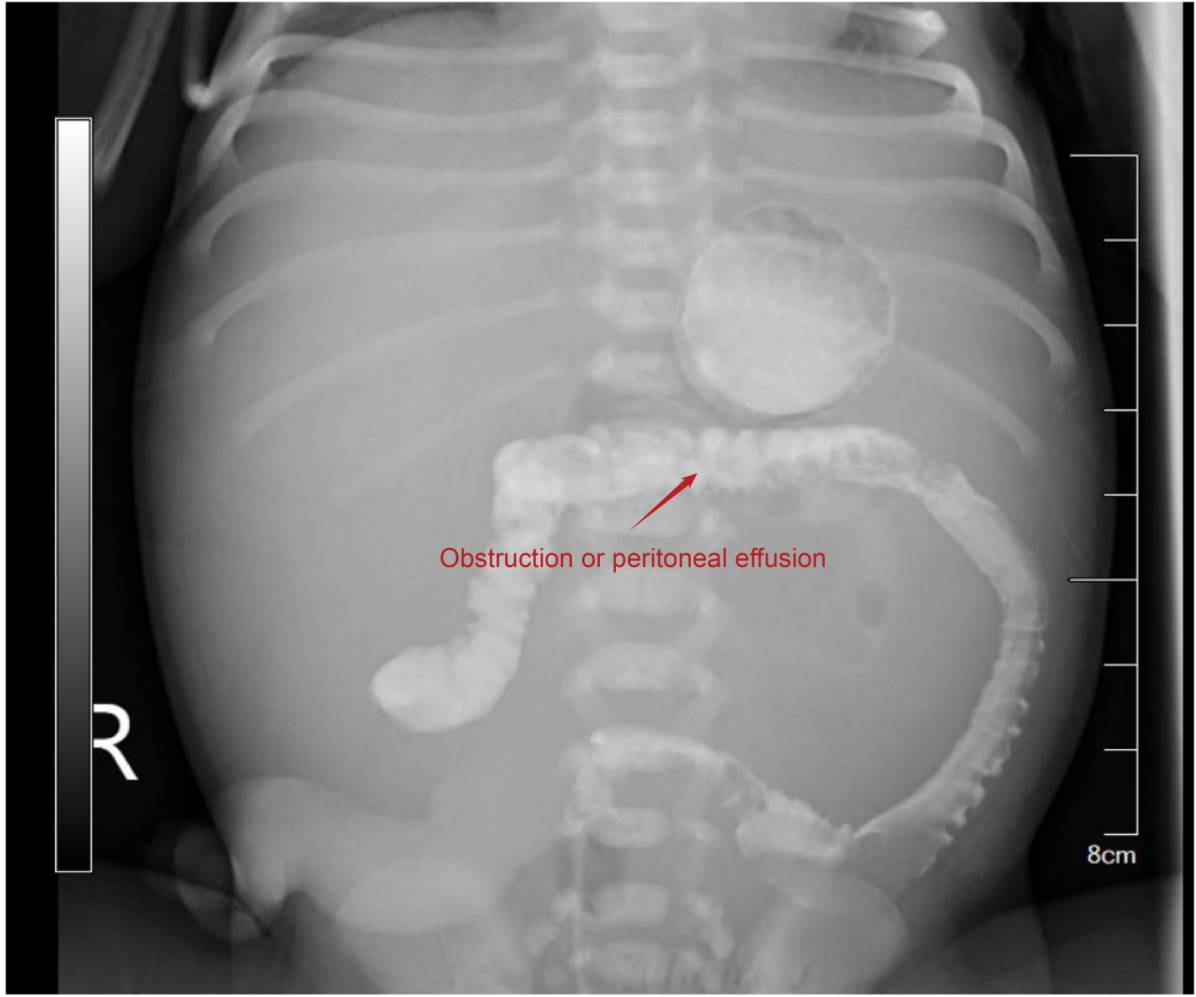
Abdominal x-ray showing high-density imaging in the gastrointestinal region. High-density imaging suggests gastrointestinal obstruction or intra-abdominal fluid accumulation.
